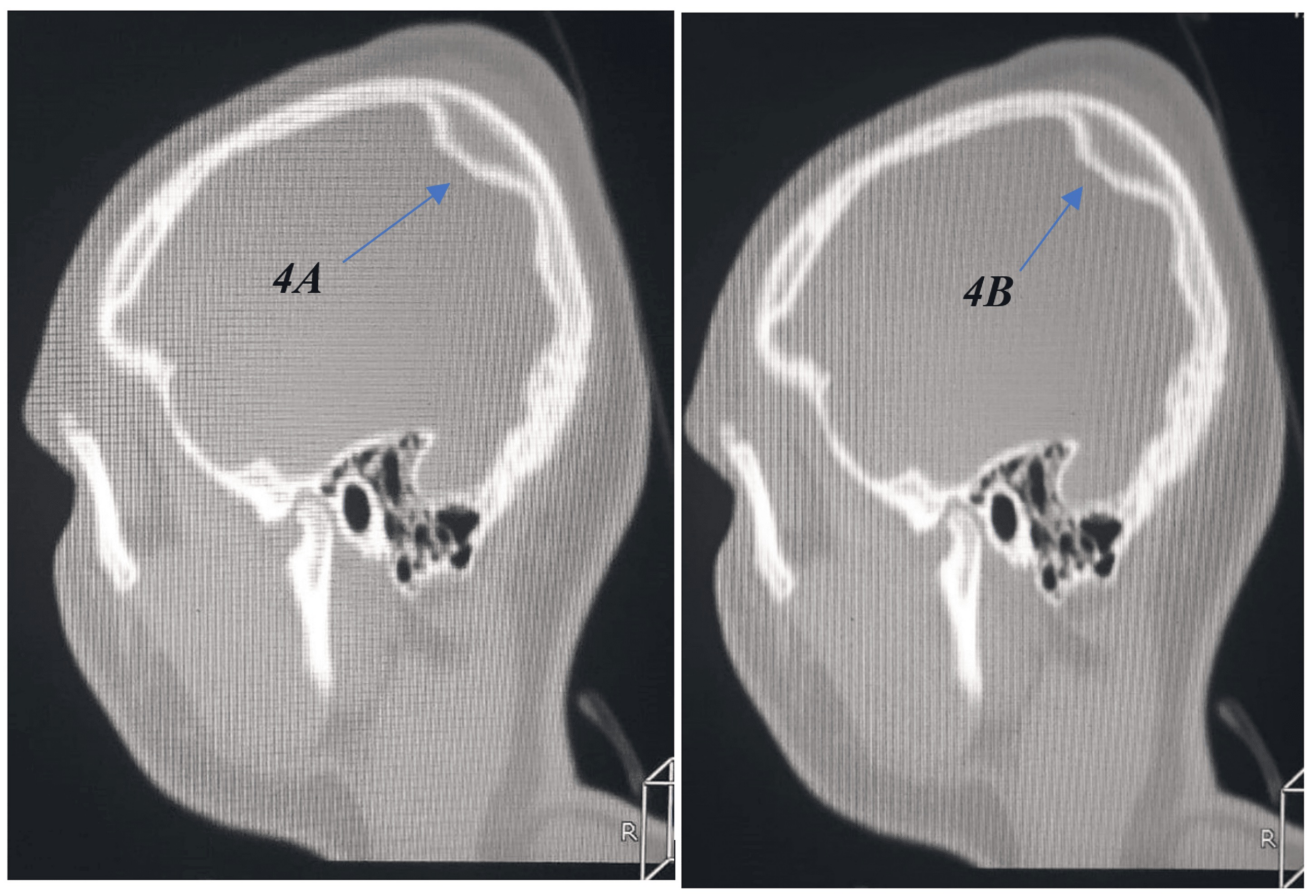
(A, B): Sagittal CT scan (bone window) further delineating the intact outer table and the subtle depression of the inner table.
